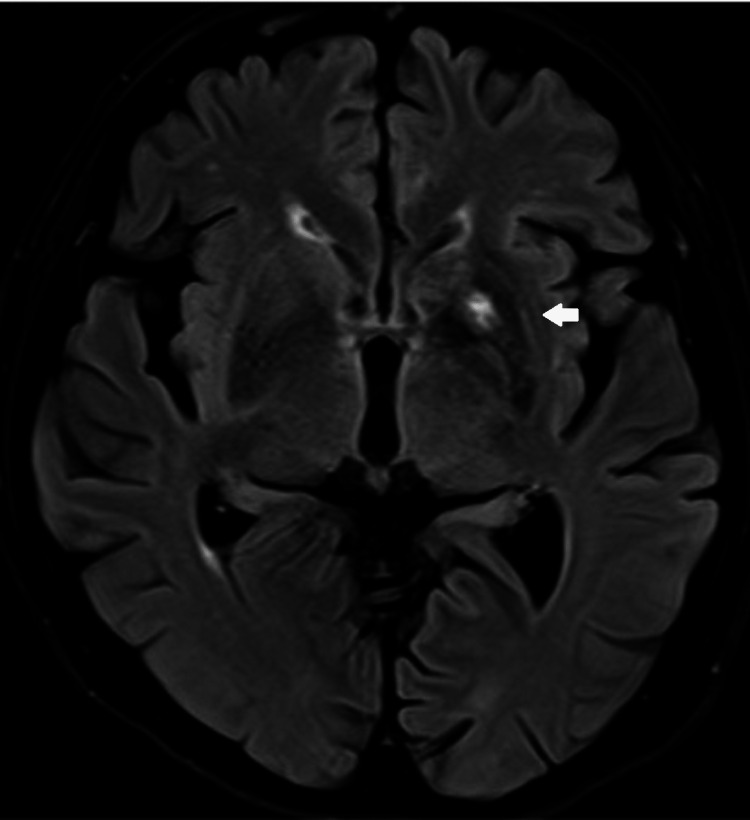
MRI Brain - Focal fluid attenuated inversion recovery (FLAIR) hyperintensity at the left lentiform nucleus
